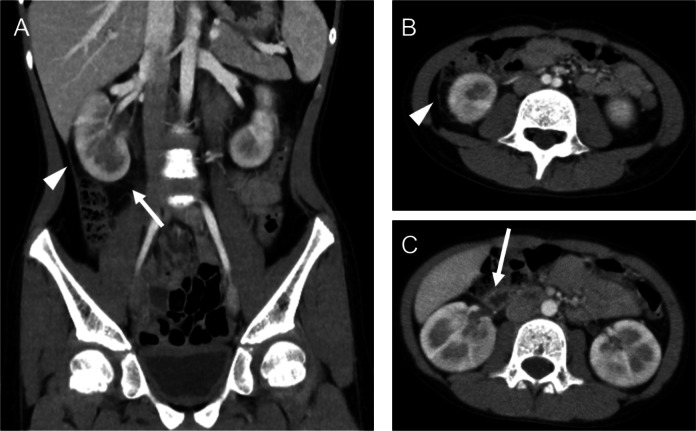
Contrast-enhanced abdominal CT image of the patient. A Coronal contrast-enhanced abdominal CT scan of the patient. The arrowhead indicates peritoneal thickening in the right paracolic gutter, while the arrow denotes fat stranding around the right kidney. B Axial contrast-enhanced abdominal CT scan of the patient. The arrowhead shows peritoneal thickening in the right paracolic gutter. C Axial contrast-enhanced abdominal CT scan of the patient. The duodenal wall appears thickened (arrow)
